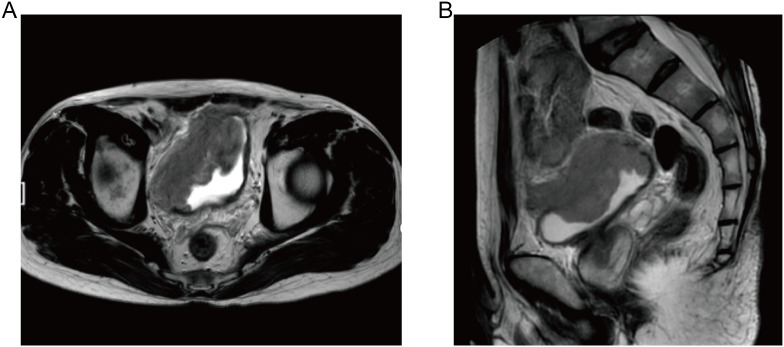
Pelvic MRI of the patient at diagnosis. (A) Axial T2-weighted imaging (T2WI) shows a space-occupying lesion in the right anterior and right lateral walls of the bladder with slightly high signal relative to the bladder wall. (B) Sagittal T2WI reveals a slightly high signal within the bladder compared with the bladder wall, with invasion of the perivesical fat space.
